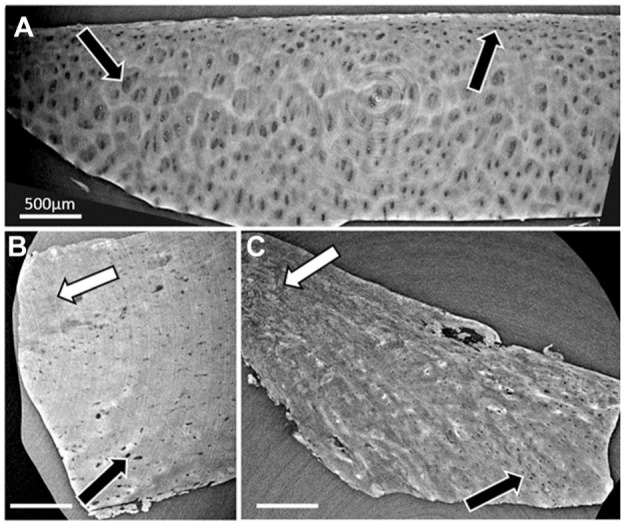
Representative computed tomography slices of (A) a control sample and (B, C) 2 morphologically distinctly different FLAP samples. Black arrows mark chondrocyte lacunae, and bright arrows mark cell-free areas with a fibrous appearance. In all images, the superficial cartilage surface is oriented toward the top.
